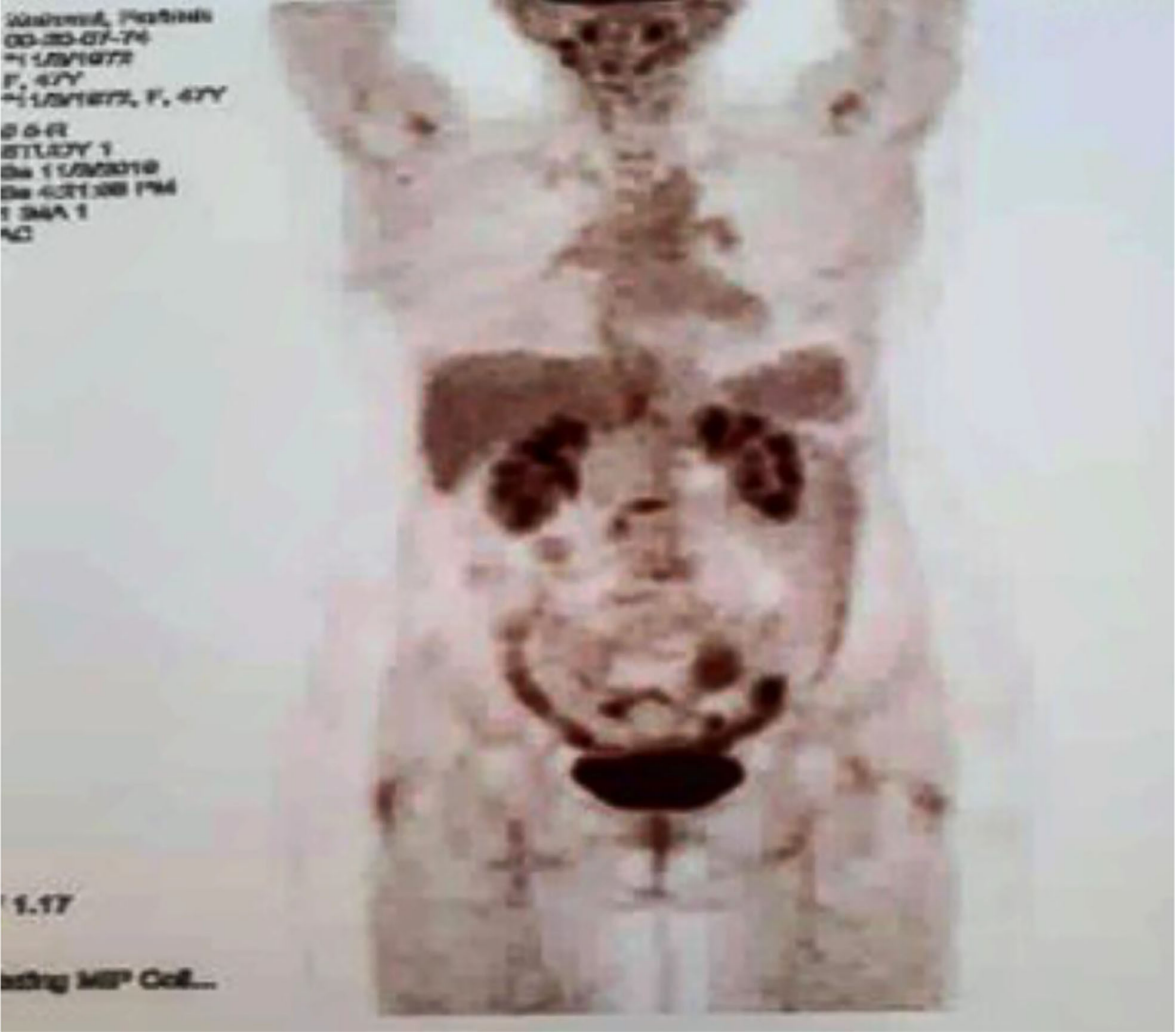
Third PET/CT on 03 November 2019. No metabolic evidence of malignancy in the images of the target parts of the body, which was compatible with continued remission.
